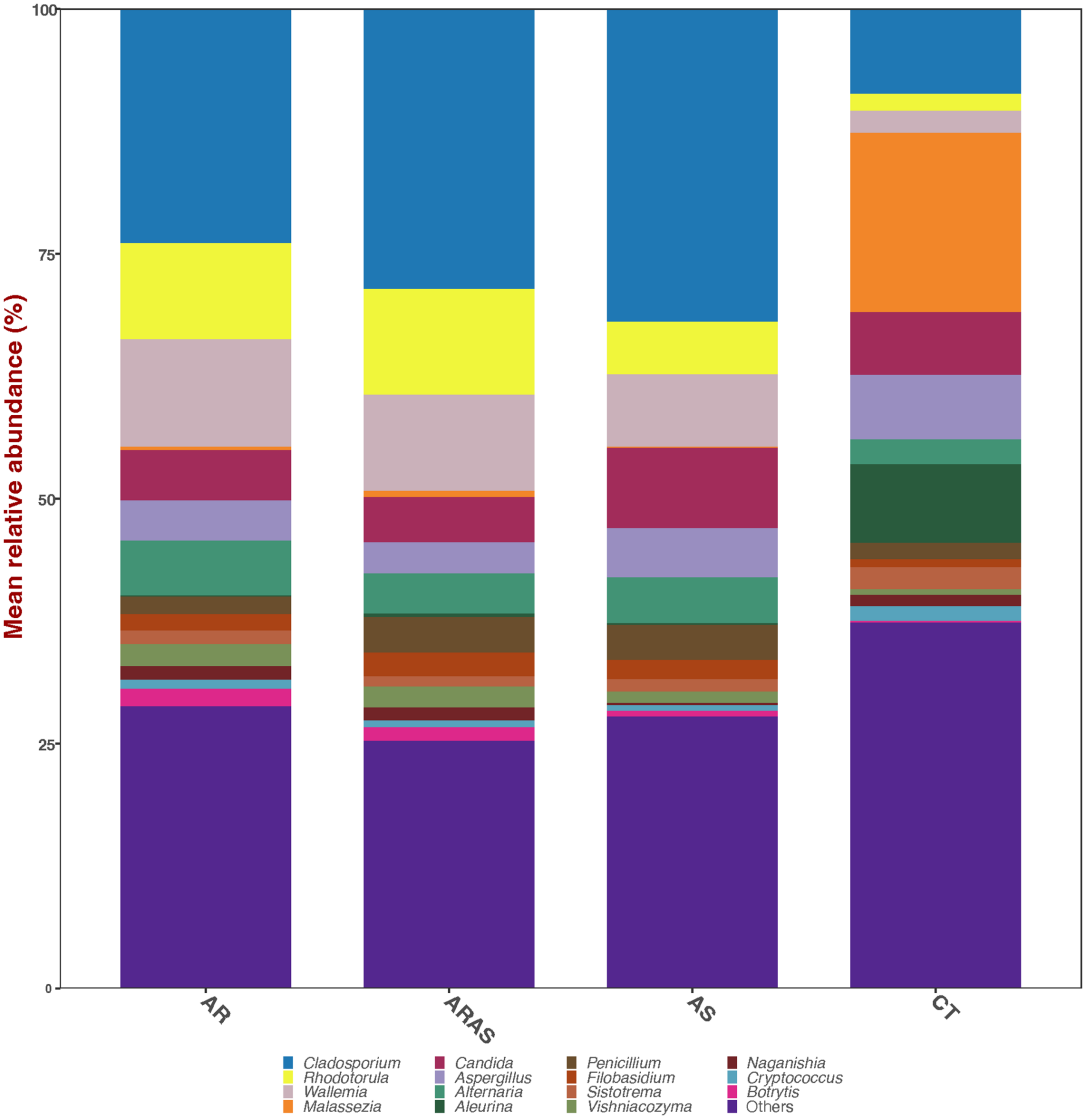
Alpha-diversity estimates (Chao1, Shannon, ACE, and phylogenetic diversity) and statistical significance (linear model test) in nasal fungal communities from participants with allergic rhinitis (AR), AR with comorbid asthma (ARAS), asthma (AS), and healthy controls (CT). ns, not significant; p* ≤ 0.05; *p* ≤ 0.0001.
